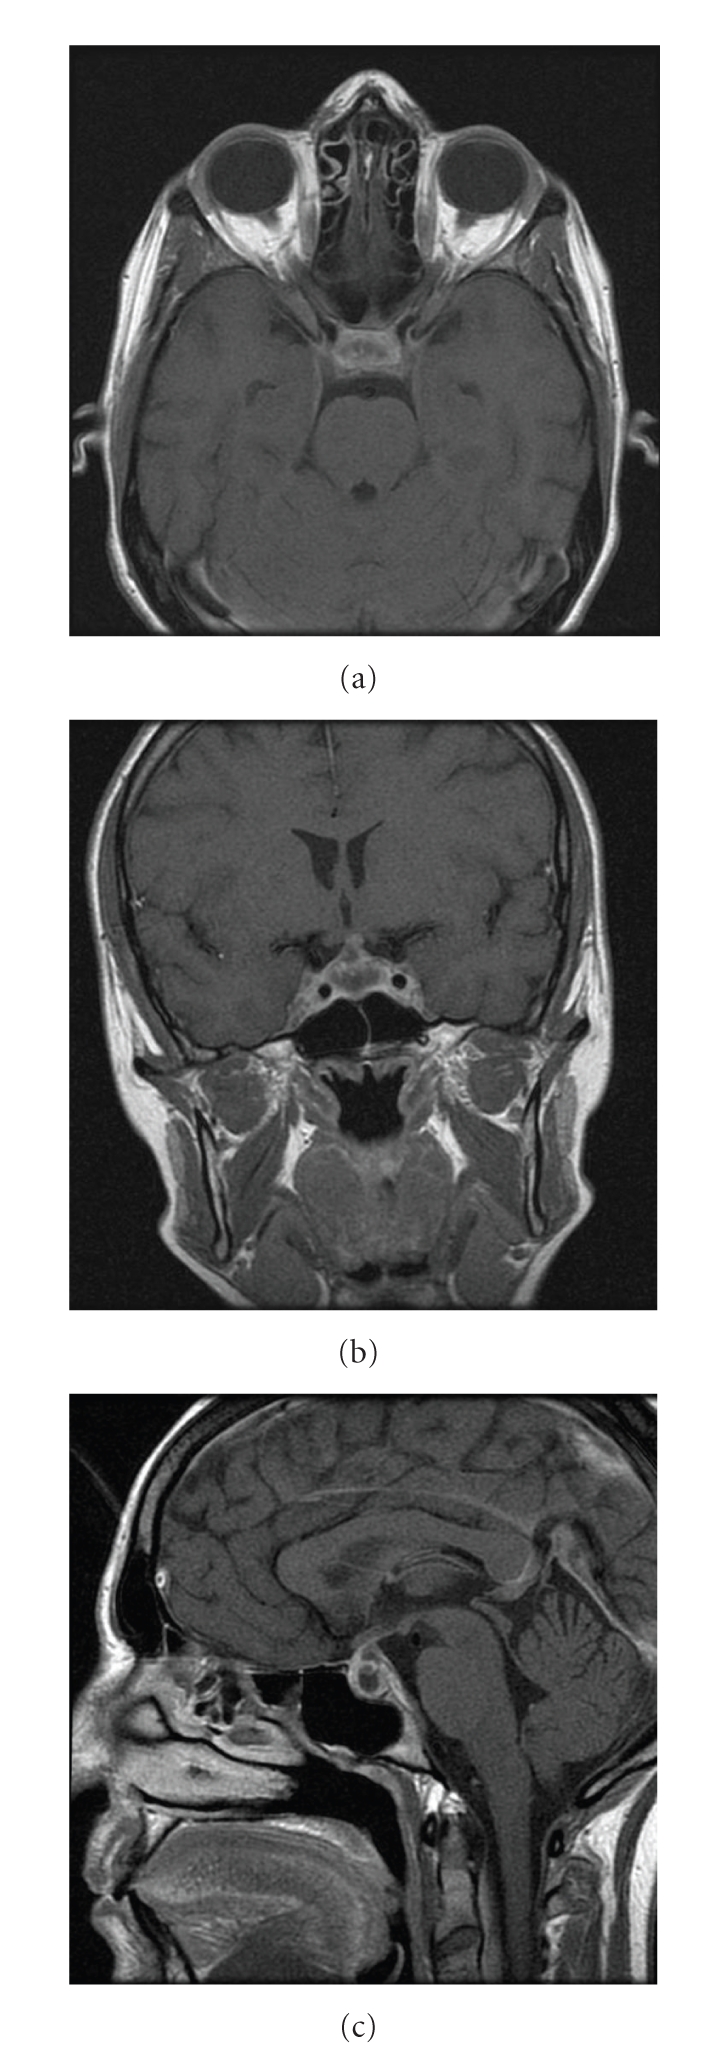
Pre-operative axial (a), coronal (b) and sagittal (c) Gadolinium-enhanced T1-w MR images showing the cystic lesion in the sella.
